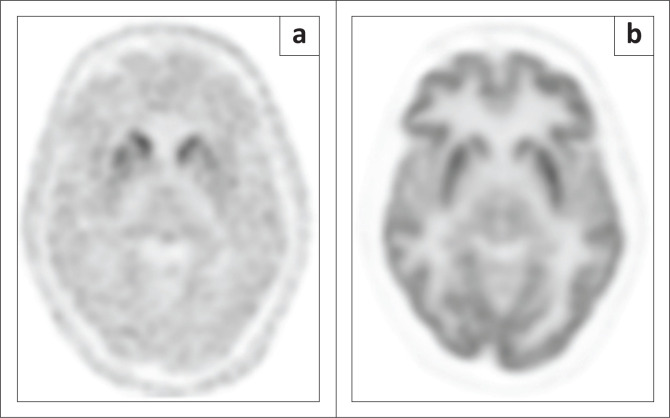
Fluorine-18 FDOPA (a) and FDG (b) PET scans in a patient with a movement disorder. The FDOPA PET convincingly demonstrates dopaminergic loss in bilateral putamina, while the FDG study demonstrates normal metabolism. These features are consistent with a diagnosis of idiopathic Parkinson’s disease.
